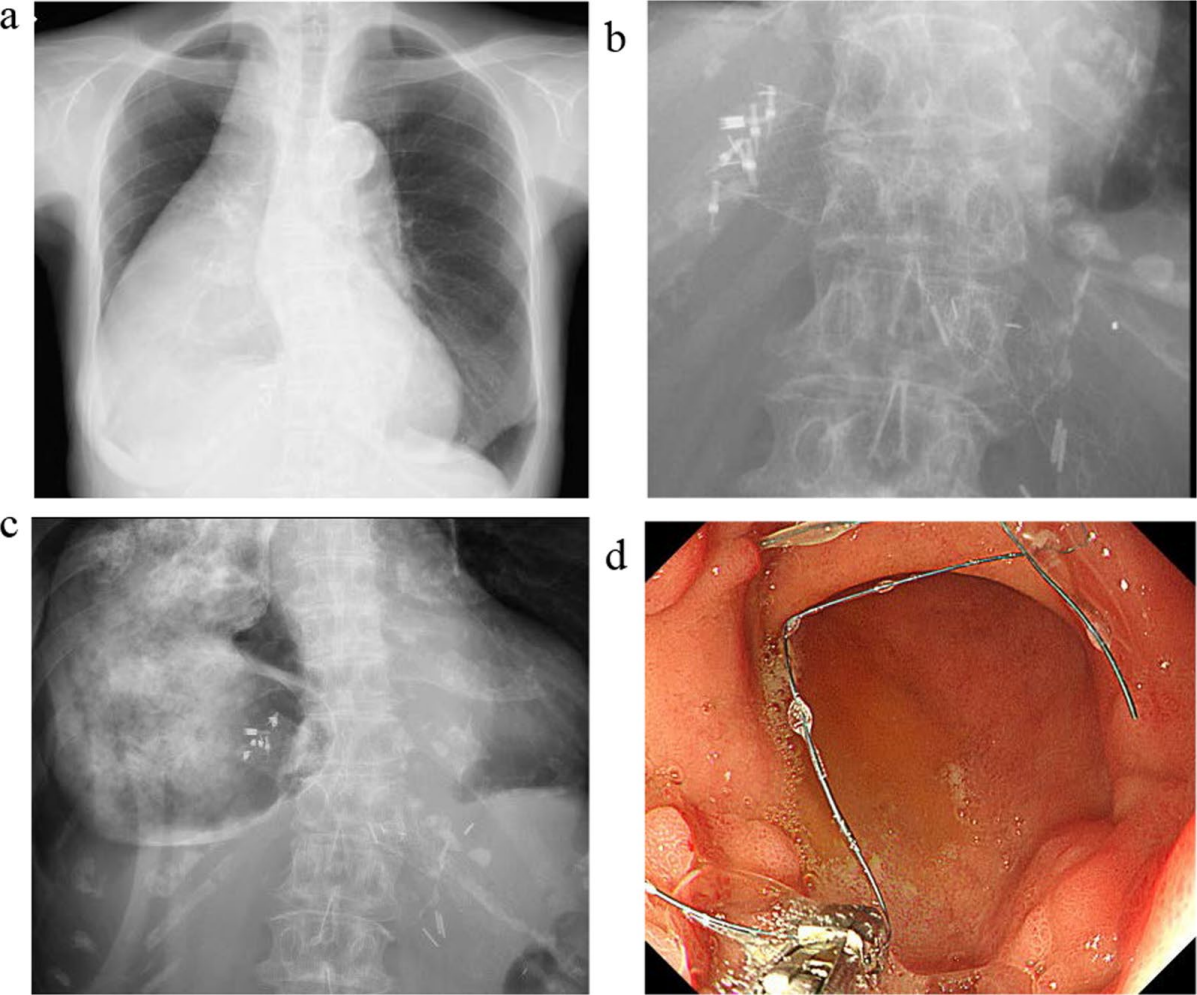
Examination findings when the patient complained of vomiting recurrence 2 years postoperatively. a A dilated gastric tube is visible in the thoracic cavity on chest X-ray. b The previously placed stent was well-fixed but had collapsed distally. c Contrast medium is retained in the thoracic part of the gastric tube on fluoroscopy. d The distally collapsed stent is visible on endoscopy
